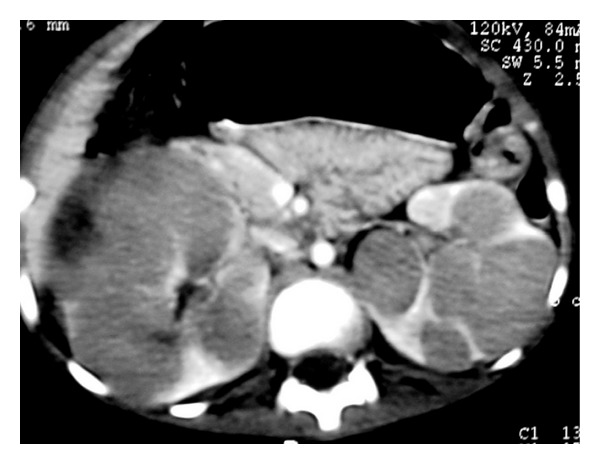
Burkitt lymphoma. Axial contrast-enhanced abdominal CT demonstrates multiple hypodense lesions involving the kidneys.
